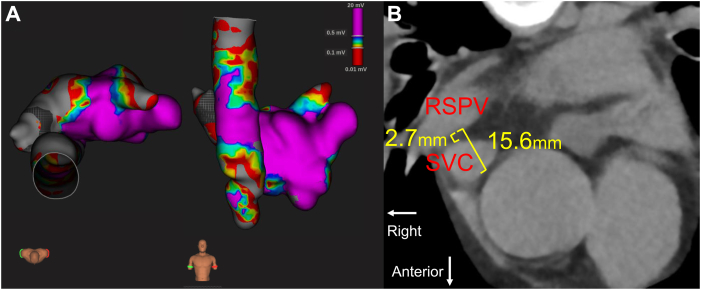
A: Voltage mapping of RA and LA during AT. The SVC is located in front of the upper branch of RSPV. A low-voltage area was identified in the anterior-septal SVC. B: CT findings. The distance between the RSPV and SVC was 2.7 mm posteriorly and 15.6 mm anteriorly. AT = atrial tachycardia; CT = computed tomography; LA = left atrium; RA = right atrium; RSPV = right superior pulmonary vein; SVC = superior vena cava.
